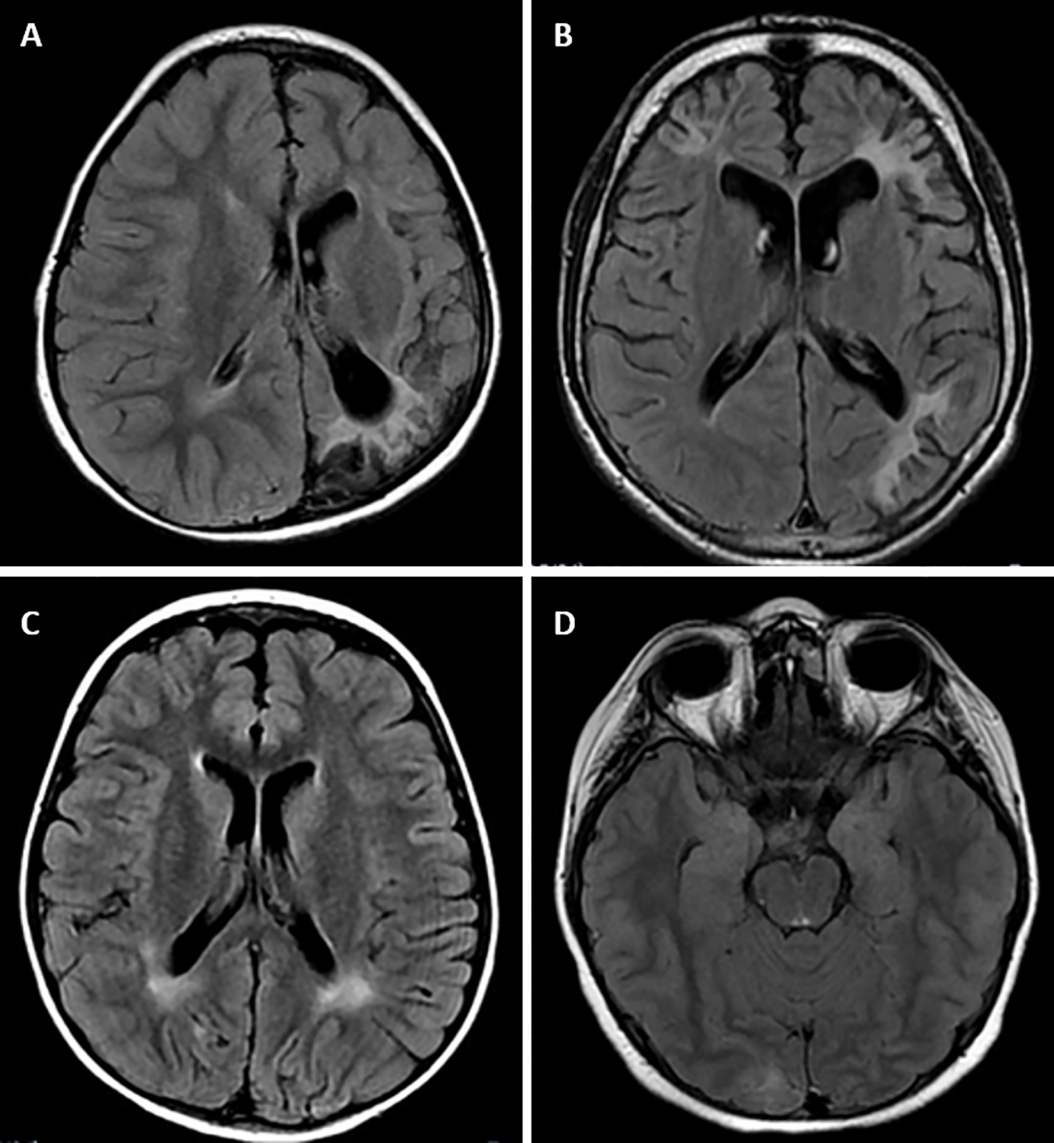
Magnetic resonance imaging alterations in patients with GSD Ia. (A) (Patient #1). Extensive areas of gliosis and encephalomalacia are observed, particularly in the cortical and subcortical areas of the left occipital and parietal lobes, as well as along the frontoparietal transition with high convexity and compensatory ectasia of corresponding portions of the ipsilateral lateral ventricle. Moreover, lesion foci are observed, which are probably related to hemosiderin deposits, extending to the posterior aspects of the nucleocapsular region with signs of chronic Wallerian degeneration of the corresponding cortico-spinal tract. There is substantial left-sided cerebral atrophy. (B) (Patient #12) Retracted lesions affecting the cortex and subcortical white matter of the bilateral frontal regions and the left parieto-temporal transition are evident. These changes are suggestive of encephalomalacia and vascular sequelae. (C) (Patient #9) Oval foci showing high-intensity on T2-weighted sequences in the central white matter and projecting close to the peritrigonal regions. These nonspecific changes could represent gliosis around the perivascular spaces (terminal myelination areas). (D) (Patient #10) Small retracted lesions affecting the posterior occipital poles with loss of volume, especially on the right side, suggestive of lesions occuring secondary to hypoglycemia.
